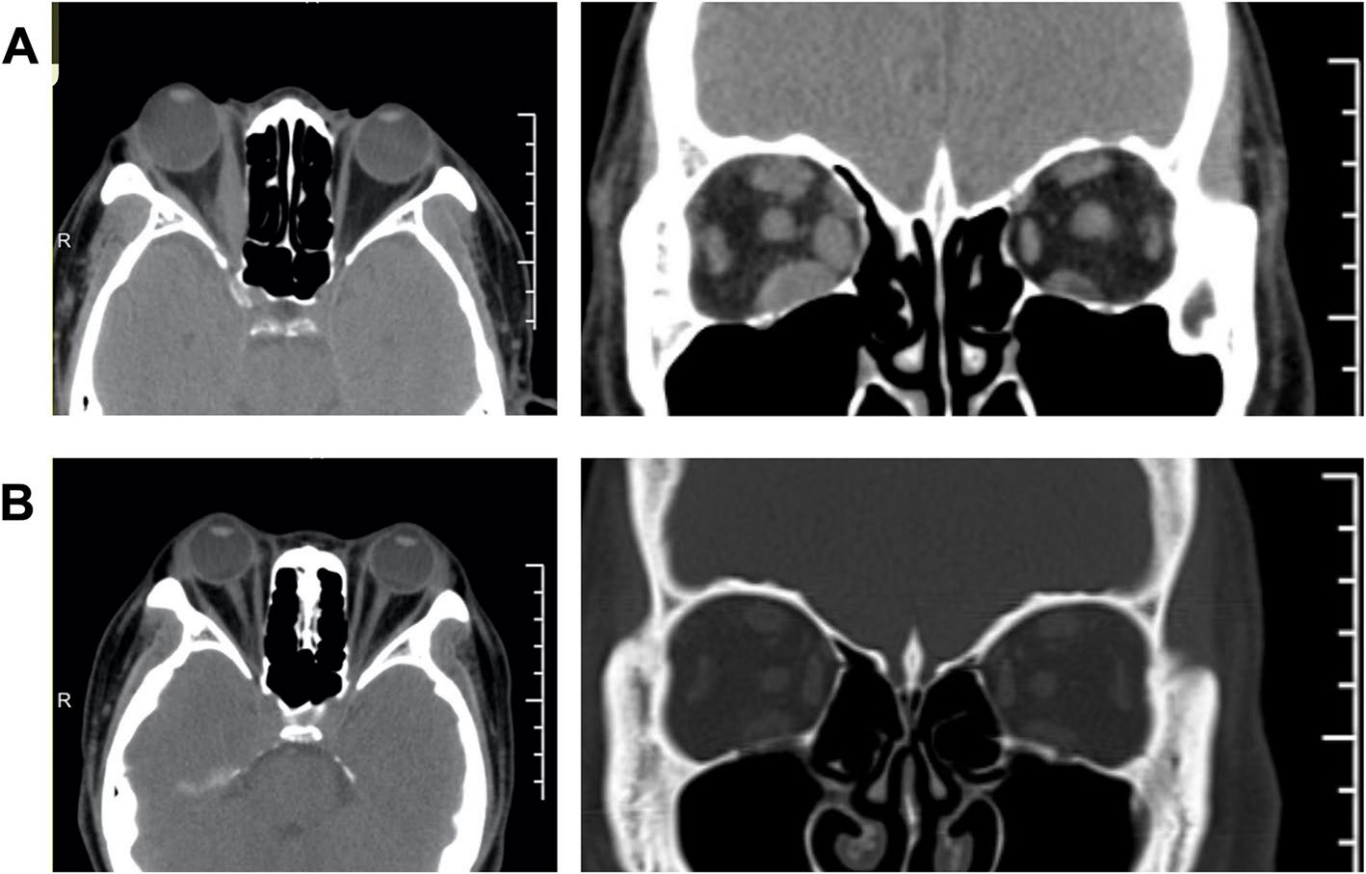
Imaging of TAO classification according to orbital CT scan. A Horizontal and coronal view of the muscle expansion type. CT scans revealed right eye protrusion, expansion of the inferior rectus, superior rectus, medial rectus muscles, and crowding of the orbital apex. B Horizontal and coronal view of the fat hyperplasia type. CT scans revealed binocular protrusion, increased orbital adipose tissue, and crowding of the orbital apex
